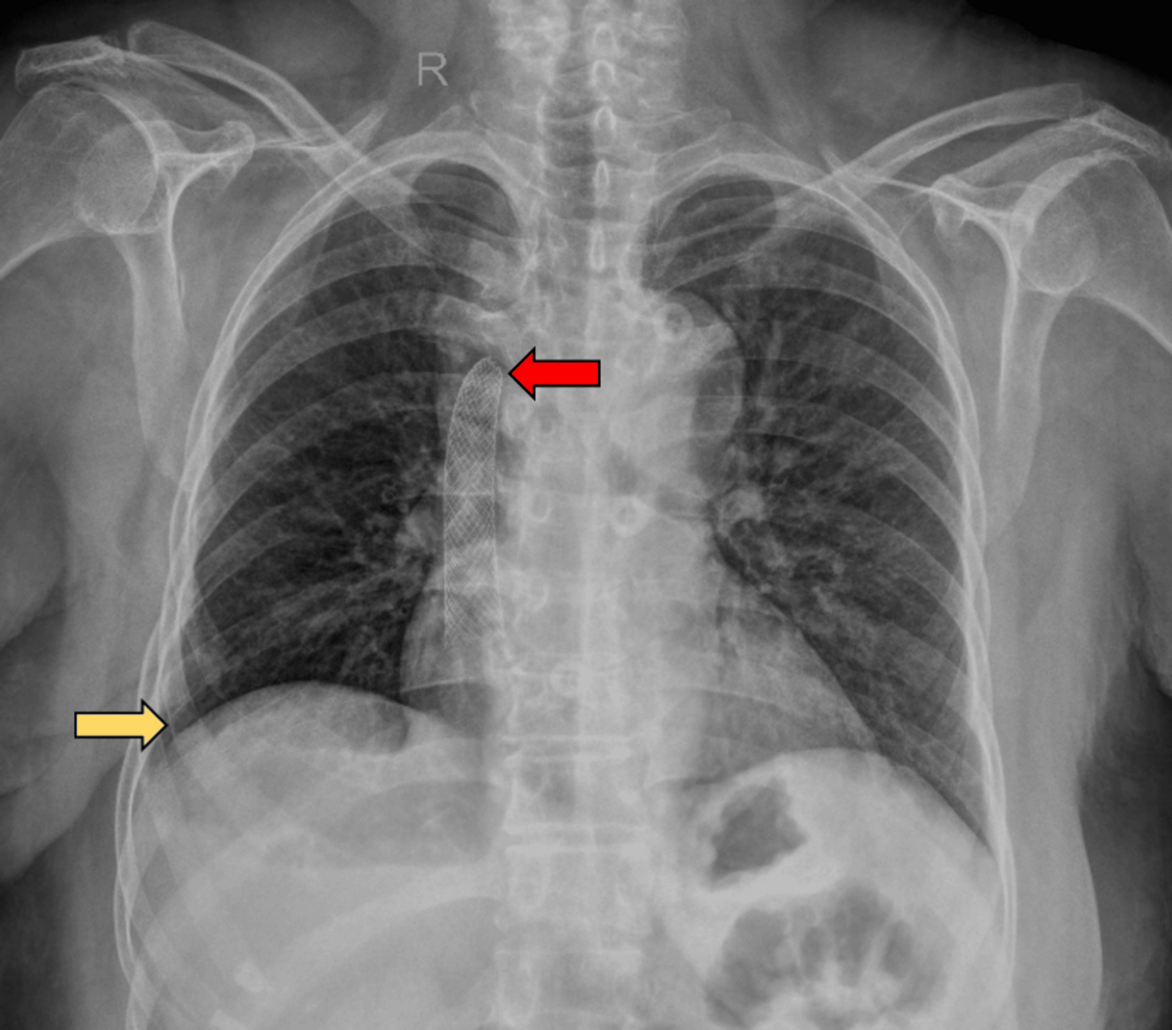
Post-procedural frontal chest radiograph showing complete resolution of pleural effusion (yellow arrow). Mild proximal stent pursing within the left brachiocephalic vein (red arrow), likely due to chronic venous fibrosis, was noted.
